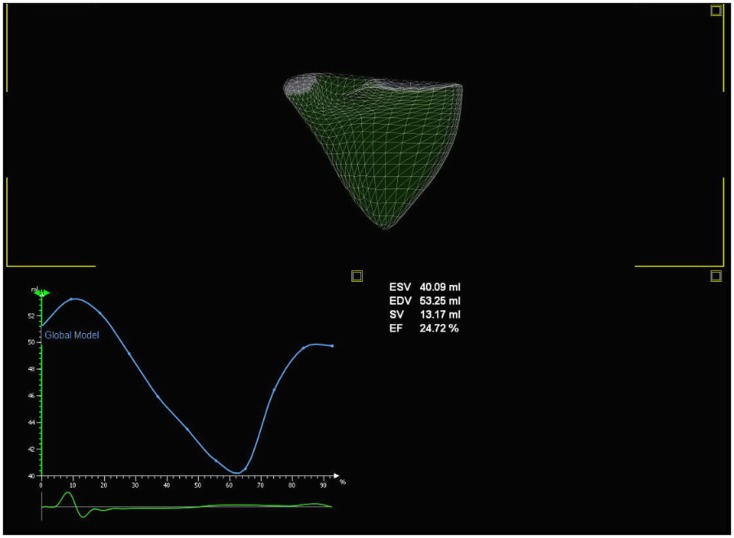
Three-dimensional echocardiography evaluation of the right heart volume and ejection fraction.
